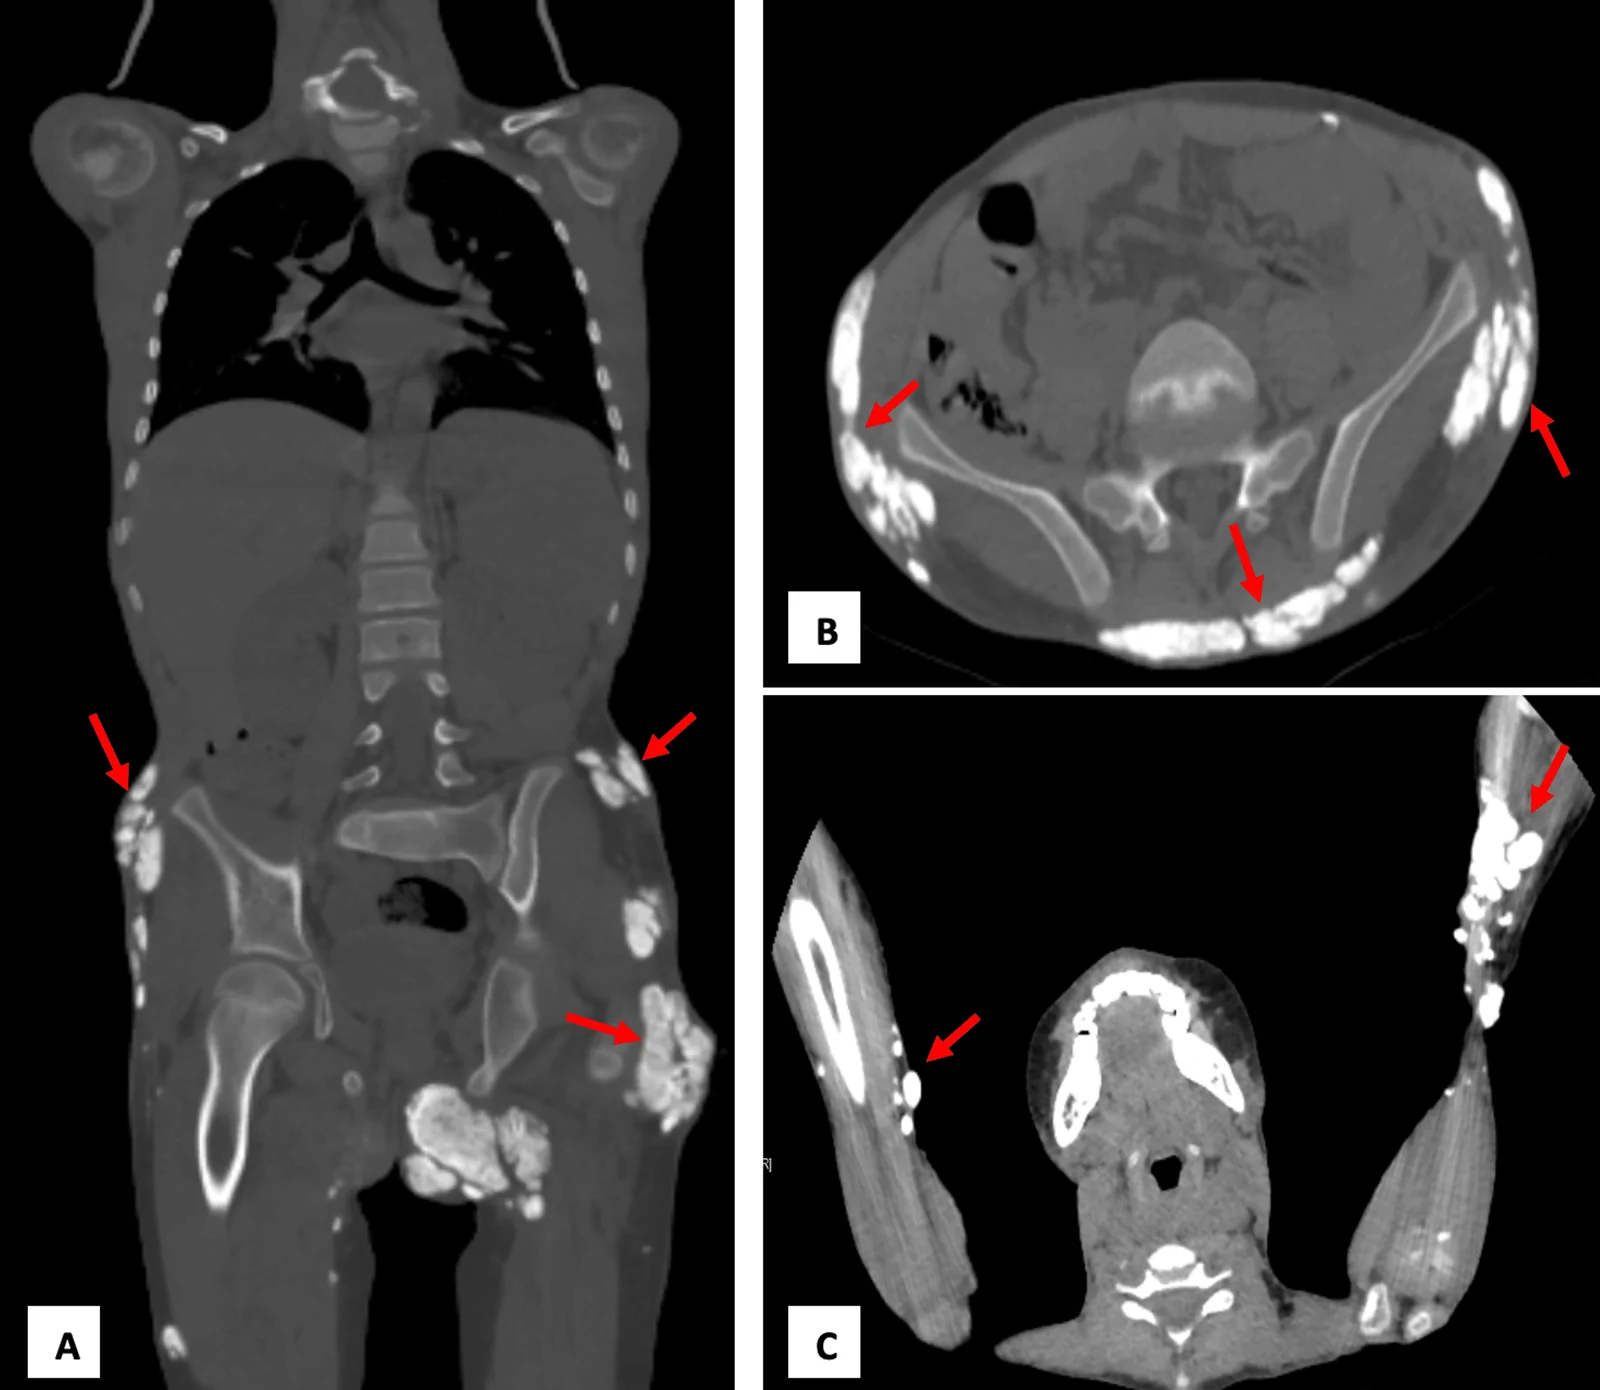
Whole-body computed tomography. (A) Coronal reconstruction showing extensive subcutaneous and periarticular soft tissue calcifications (red arrows) in the pelvic, gluteal, and lower limb regions, without visceral or thoracic involvement. (B) Axial view at the pelvic/inguinal level showing bilateral subcutaneous calcified deposits (red arrows). (C) Axial view at the level of the shoulders showing calcified soft tissue deposits of both proximal upper limbs (red arrows). No bone destruction, lymphadenopathy, or neoplastic lesion was identified.
